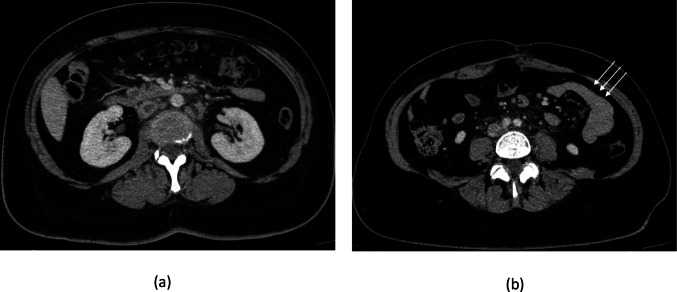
Axial contrast-enhanced abdominopelvic CT shows a central filling defect in the inferior vena cava with retroperitoneal and mesenteric root lymphadenopathy (a), and multiple collapsed jejunal loops (arrows) exhibiting mural thickening and peri-enteric fat stranding consistent with inflammatory jejunitis (b)
